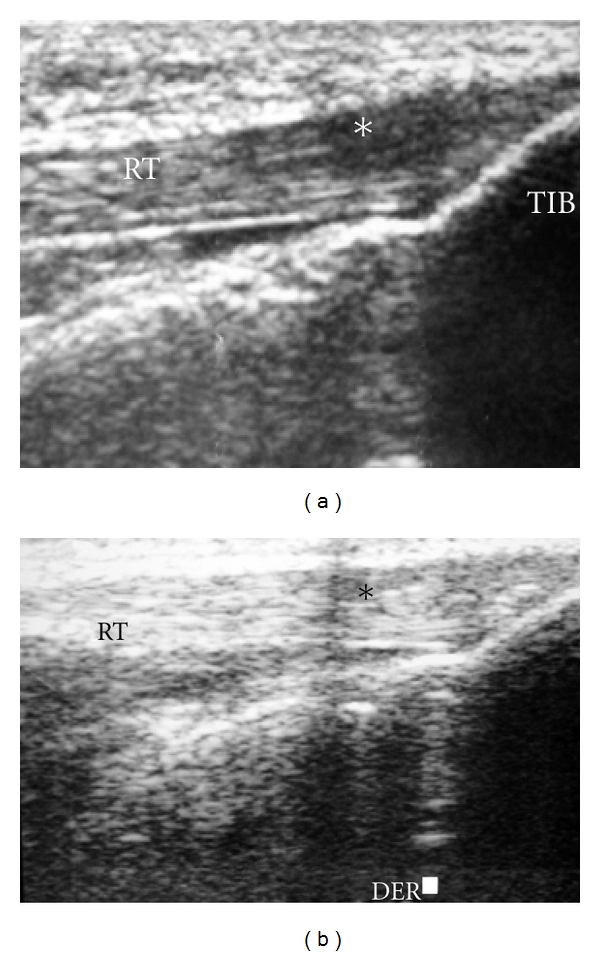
Table 1 tab1: Sonographic grading of the patellar tendinopathy. Grade ratio between injured area and whole tendon section at US scan.
